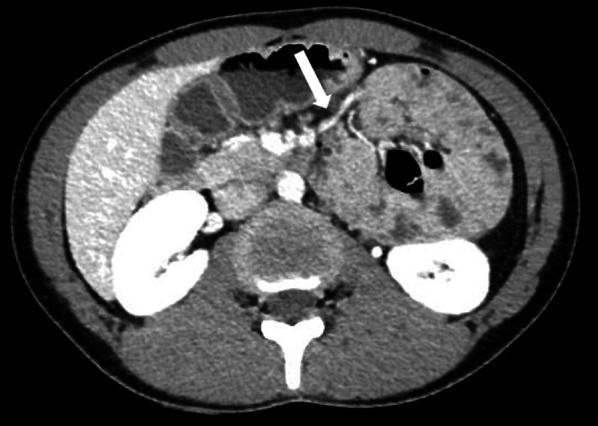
A multi-detector contrast-enhanced computed tomography scan showed no signs of ischemic findings. The small bowel was congregated on the left upper quadrant of the abdomen through the dorsal side of the inferior mesenteric vein (white arrow)
